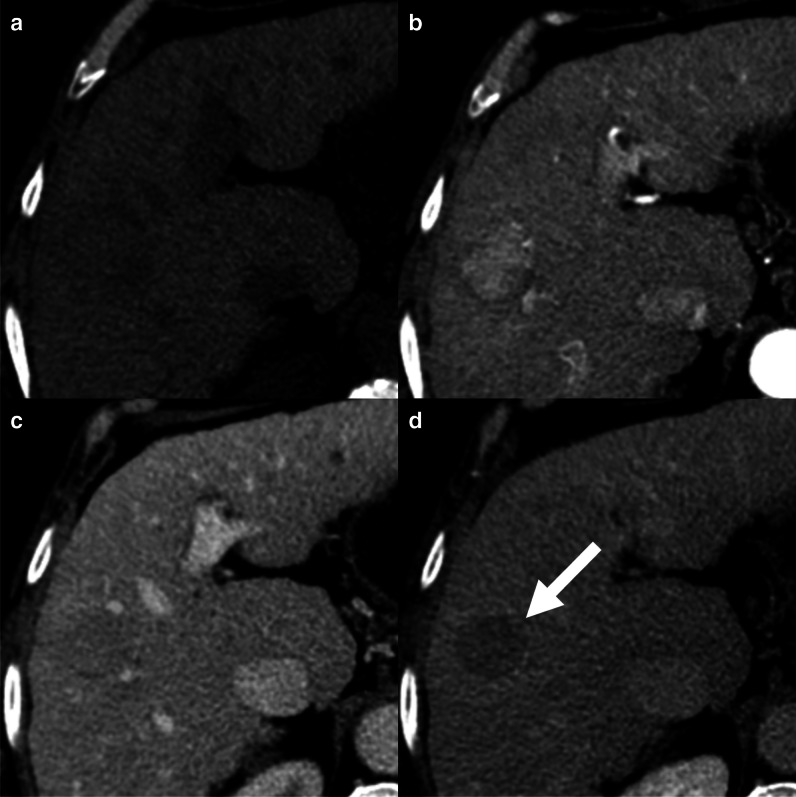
A 66-year-old woman with cirrhosis and hepatocellular carcinoma. Contrast-enhanced CT shows a lesion isodense on pre-contrast (a), with non-rim arterial phase hyperenhancement (b), isodense on portal venous phase (c) but with non-peripheral washout on delayed phase (d)
